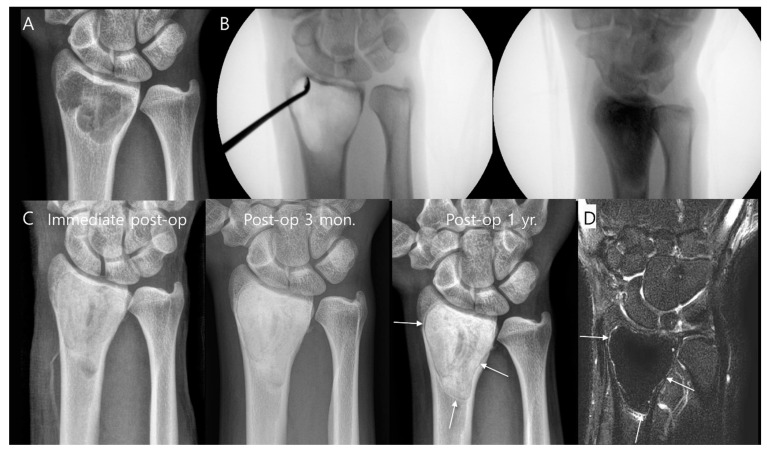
Intralesional curettage for giant cell tumor of the distal radius. (A) Preoperative plain radiograph shows a well-defined osteolytic lesion located in the epi-metaphysis of the distal radius. (B) Intraoperative fluoroscopy shows extended curettage with adjuvant treatment and cementation. (C) Postoperative serial plain radiographs demonstrate a thin, radiolucent line around the cement implant 1 year after surgery (arrows). (D) Postoperative coronal T2-weighted fat-suppressed MR image also shows a thin, hyperintense rim around the cement implant (arrows), referred to as a “clear zone”.
